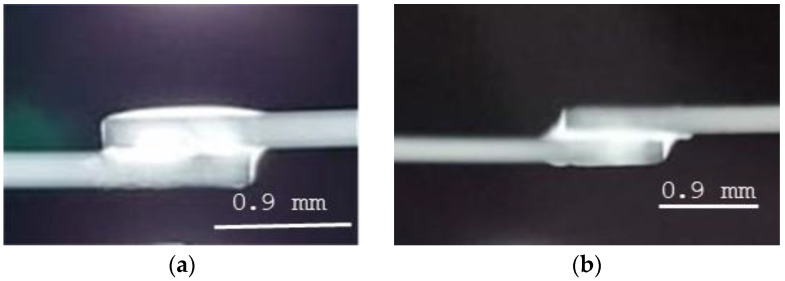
X-ray results of a solder joint (a) with a solder gap of (a) 0.1 mm and (b) 0.3 mm.
